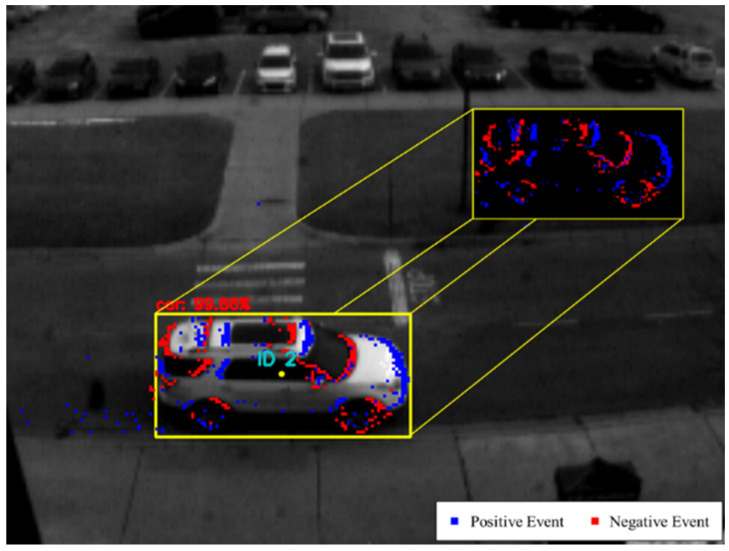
The figure demonstrates when an event mask is generated by accumulating the events located within the bounding box, as shown in the top right corner. In this frame, a white SUV is detected, as highlighted by the yellow bounding box (using the frame-based object detector SSD [39]), with 99% confidence. The tracking rate used here is 48 Hz, meaning that the window frame’s size is 21 ms, and only the events captured during this interval are displayed.
